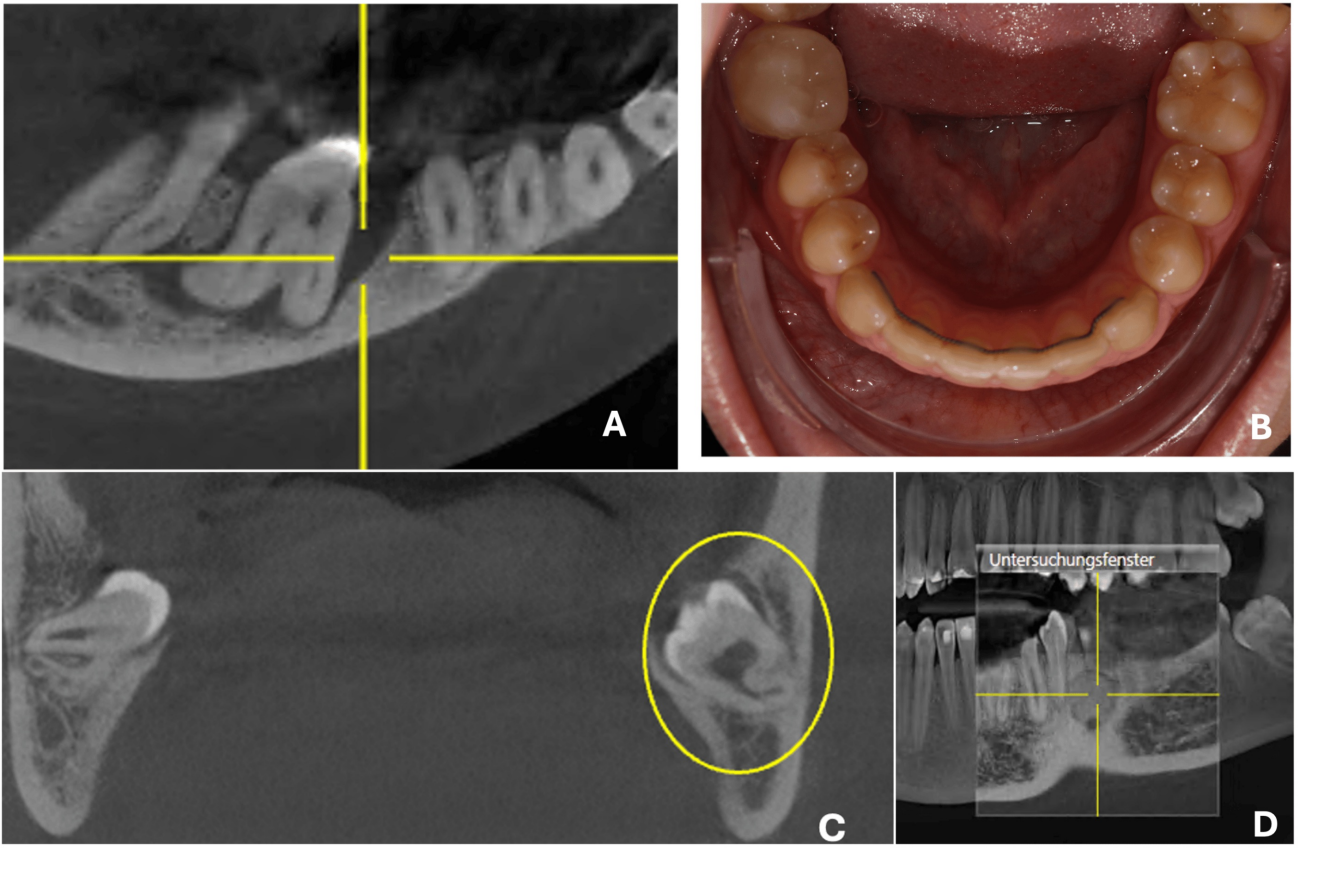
CBCT view of infectious site and donor tooth options Figure 2 shows (A) the extent of the infection of the lower right molar on the CBCT scan, (B) the intraoral photograph of the lower arch, (C) both wisdom teeth, from which the lower left was selected as the donor tooth due to its less advanced root formation, and (D) an incidental finding of a spherical radiopaque mass in the region of the lower left premolars. CBCT, cone-beam computed tomography.
